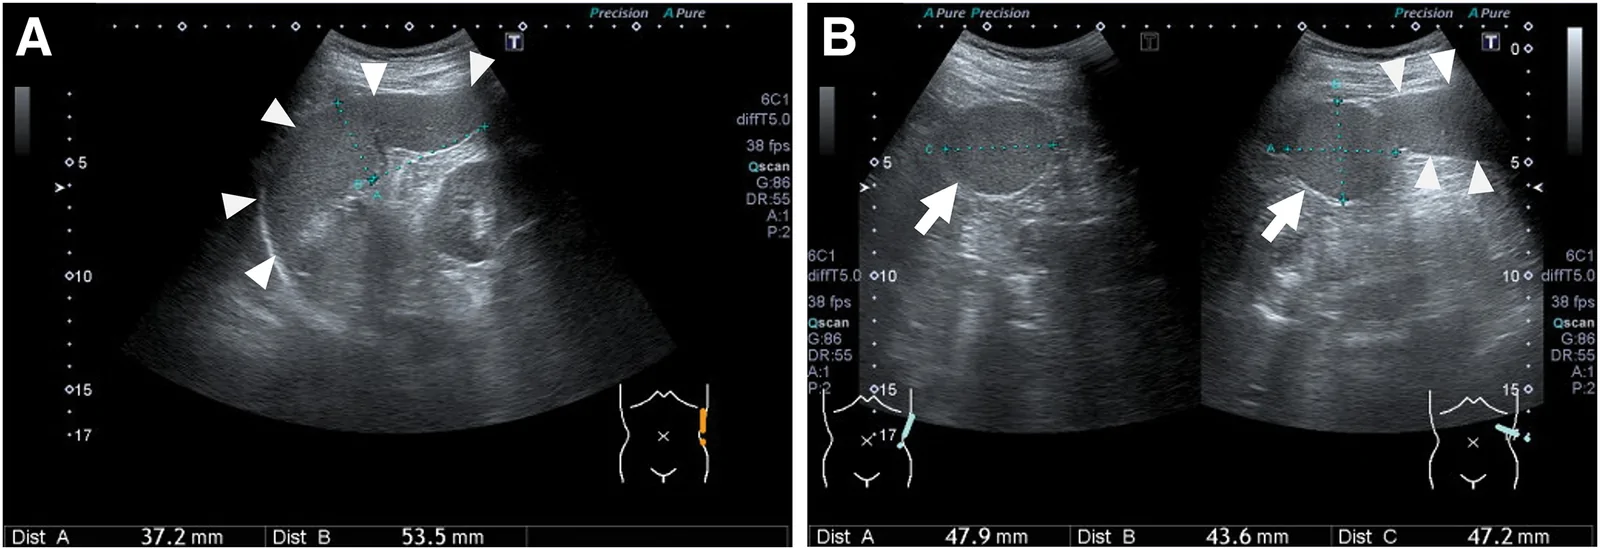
US findings at X–8 years. (A) Mild splenomegaly was detected in the left upper abdomen (arrowheads) at the time of the initial diagnosis of ET. (B) A 4.8-cm well-defined round mass was detected (arrow) adjacent to the native spleen (arrowheads). The mass was considered as an accessory spleen. ET, essential thrombocythemia; US, ultrasonography
